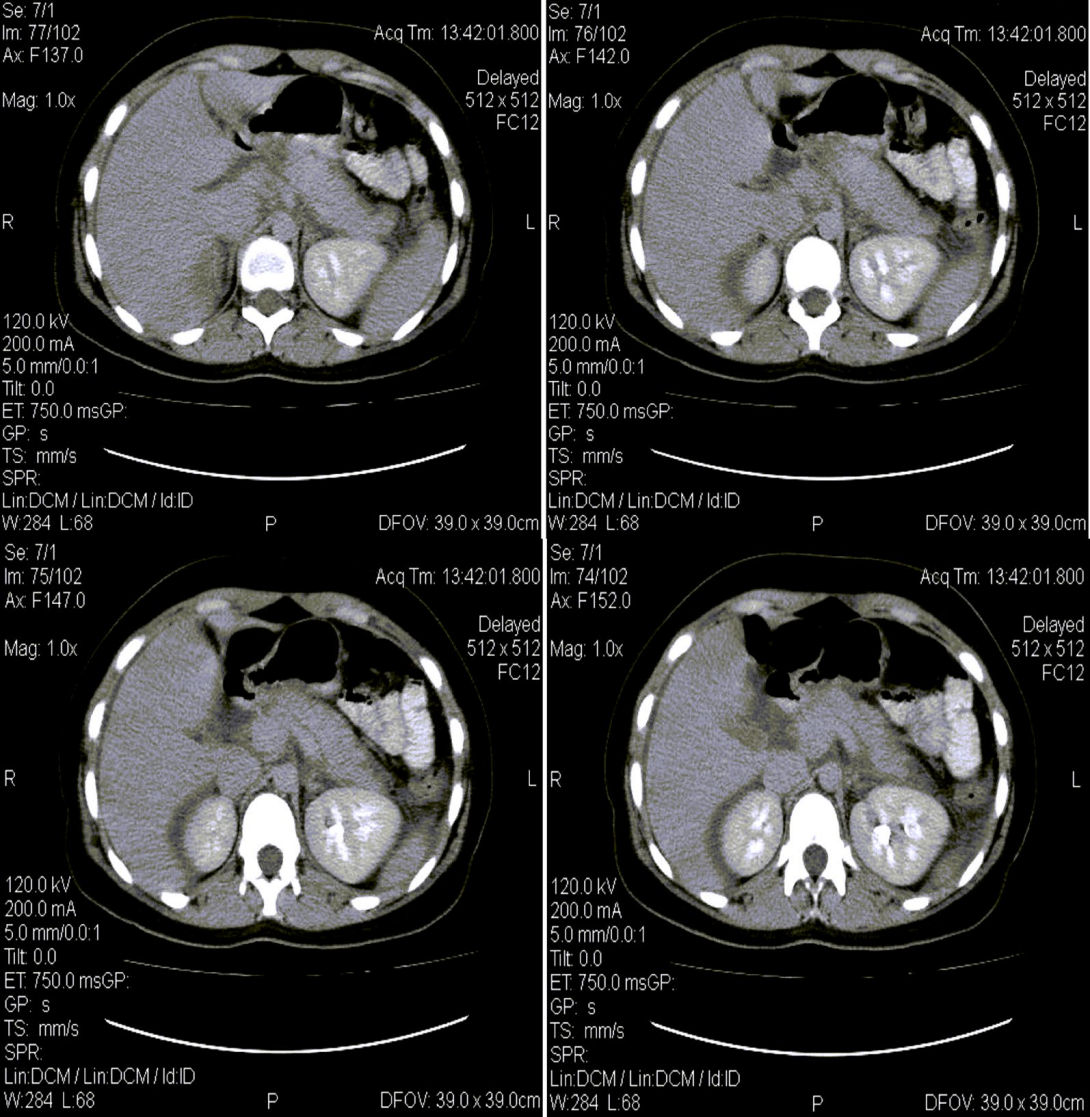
Computed tomography scan of the abdomen showing diffuse enlargement of the pancreas
